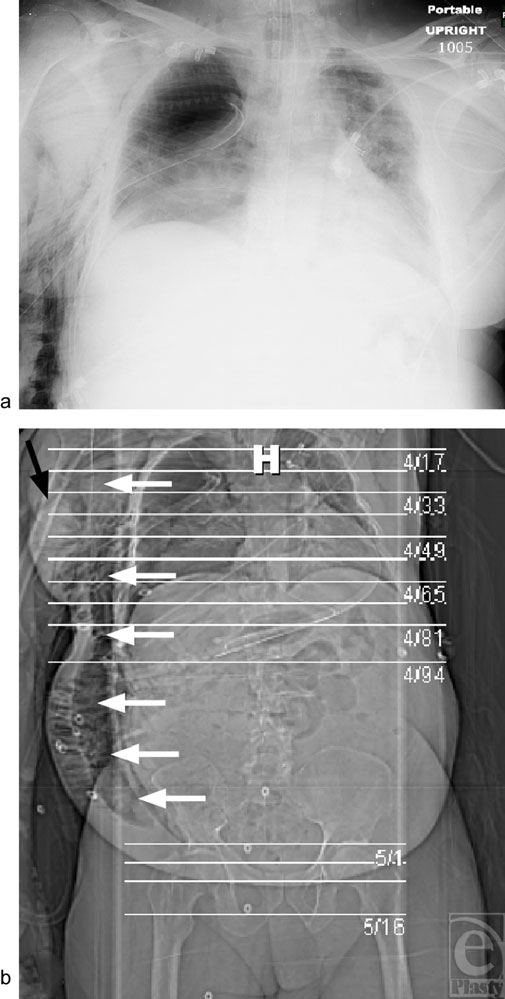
(*a*) Portable roentogram shortly after right tube thoracostomy following repeated unsuccessful attempts at needle thoracostomy demonstrates rapid development of extensive subcutaneous emphysema in conjunction with positive pressure ventilation and persistent chest tube air leak. (*b*) Computed tomography scout film approximately 12 hours after tube thoracostomy demonstrates wide extension and severe degree of subcutaneous emphysema (white arrows). Prepectoral “blowhole” incision (black arrow).
